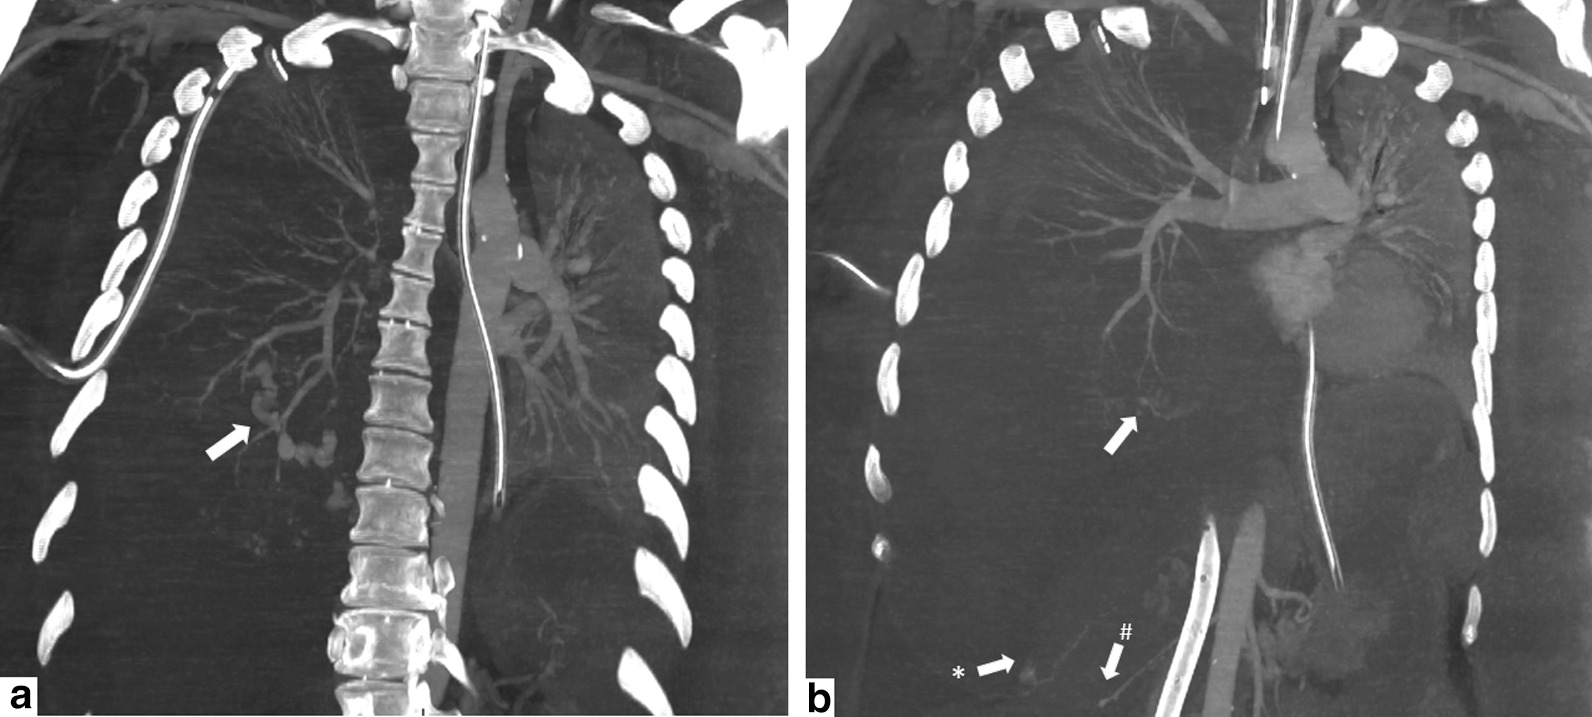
Coronal Maximum Intensity Projection (42 mm in a and 8 mm in b) chest CT images of a 46-year-old male with COVID-19 pneumonia. Note the presence of arterial pulmonary aneurysms complicated by intraparenchyma bleeding (a arrow), diaphragmatic (b star) and kidney aneurysms (b dash). An apical right pleural drain and gastric tube (panel a, b) as well as veno-venous ECMO superior and inferior canulae (panel b) can also be seen
